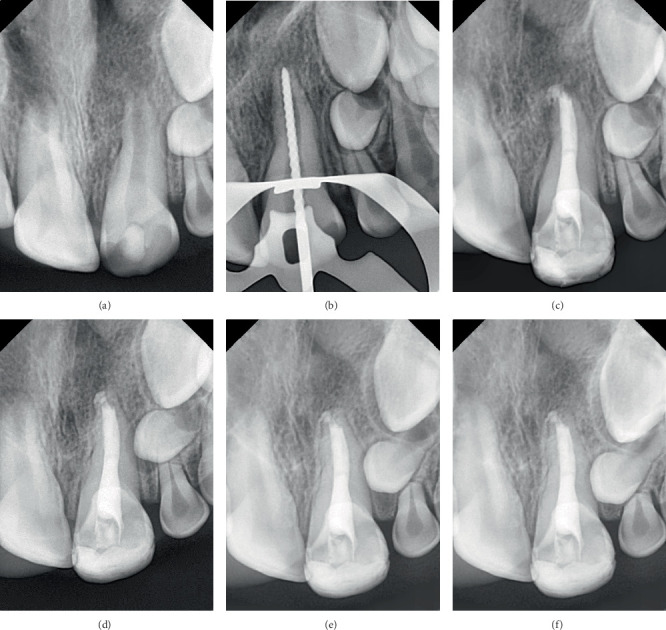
Case 3. (a) Preoperative periapical radiograph. (b) Working length radiograph. (c) Postoperative radiograph. (d) A 6-month recall radiograph. (e) A 12-month recall radiograph. (f) A 24-month recall radiograph.
